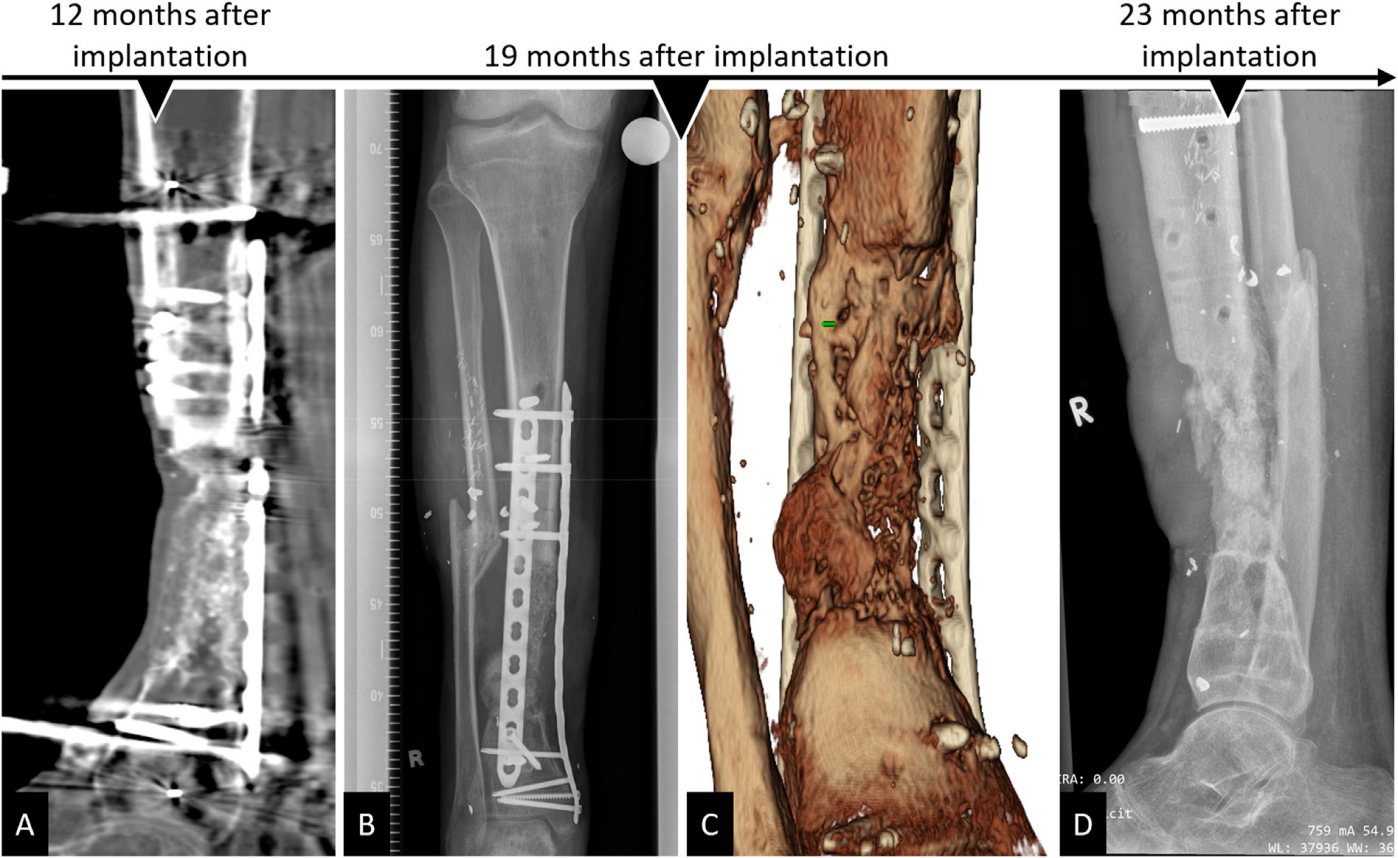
Complete bone regeneration achieved in an extra-large tibial segmental (10 ​cm) defect after implantation of an mPCL-TCP scaffold loaded with ABG and supplemented with rhBMP-2 (INFUSE® Bone Graft, Medtronic). Scaffold integration at the proximal and distal defect ends after one year (A) was observed, and an external fixator was exchanged with plate fixation. At 19 months after scaffold implantation, x-ray (B) and 3D reconstruction of the CT scan (C) showed bony fusion. At 23 months after scaffold implantation, functional reconstruction of the extra-large segmental defect was achieved and the osteosynthesis implants were removed (D).
